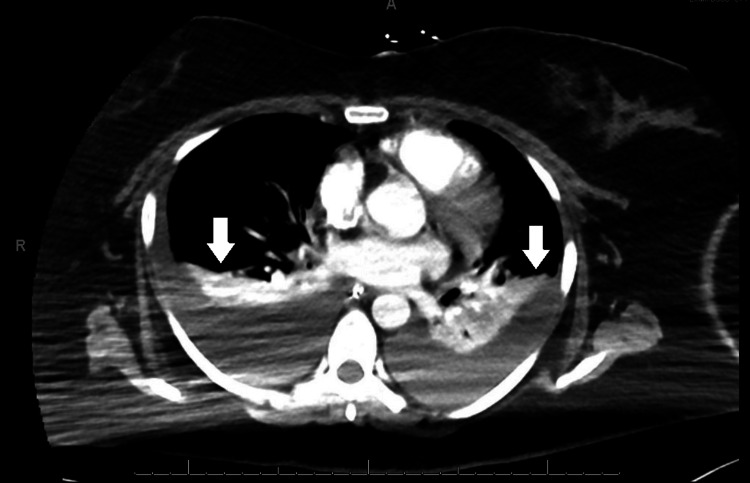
Computed tomography angiogram with arrows depicting enlarging bilateral pleural effusions.
